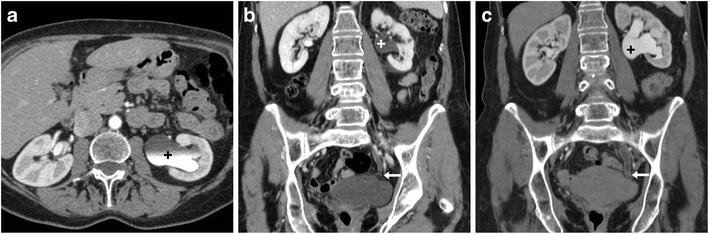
Long-term effects of an unrecognised ureteral stricture following AH 1 year earlier. Follow-up CT with biphasic contrast injection showed left-sided hydronephrosis (+) from tight ureteral stricture (arrow in C) which required endourological management
